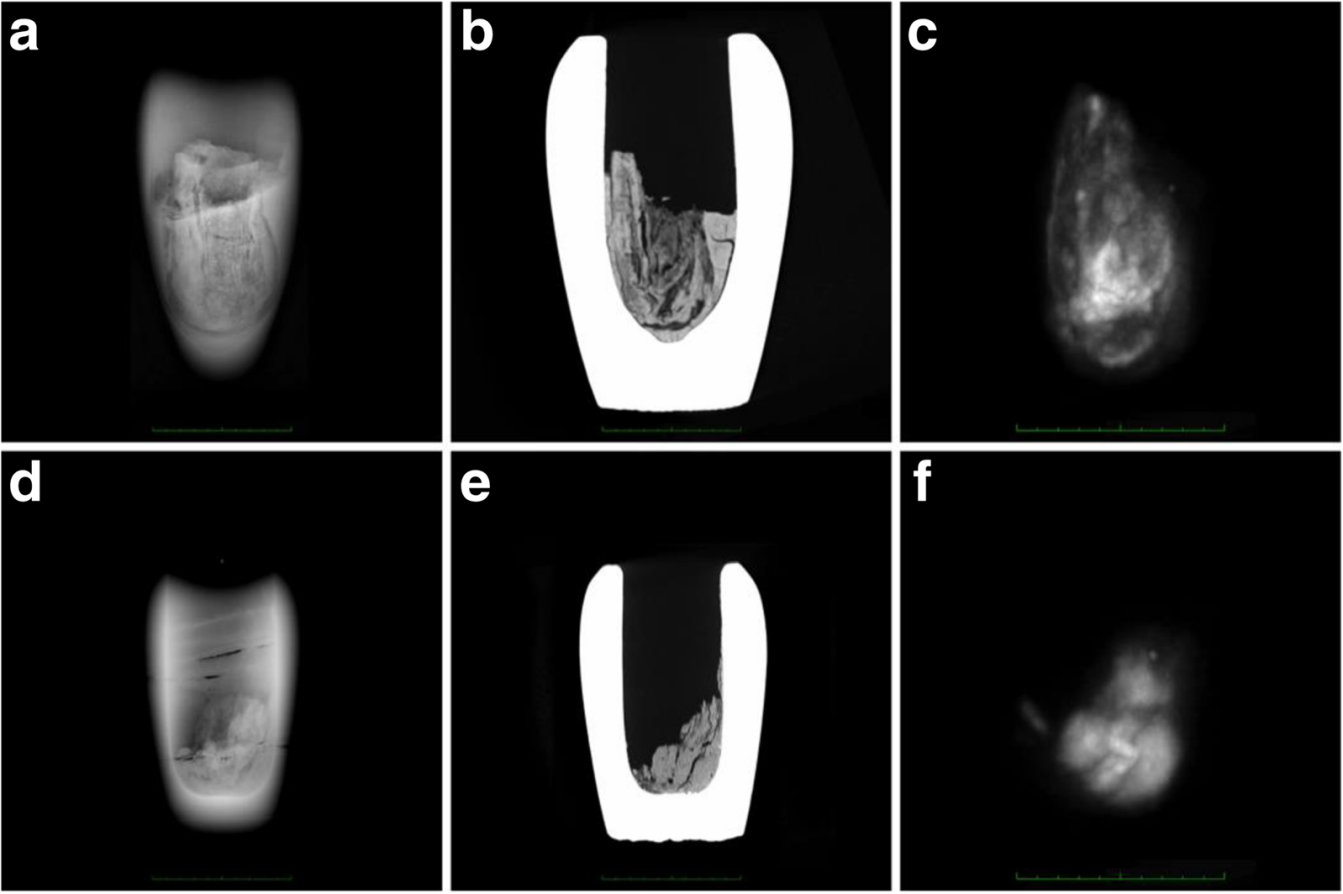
Direct comparison of the three imaging modalities: x-rays (a, d), CT (b, e) and MRI (c, f) of the canopic jars with the smallest and the largest diameter (a–c, inventory number 617; d–f, inventory number 622-1)
